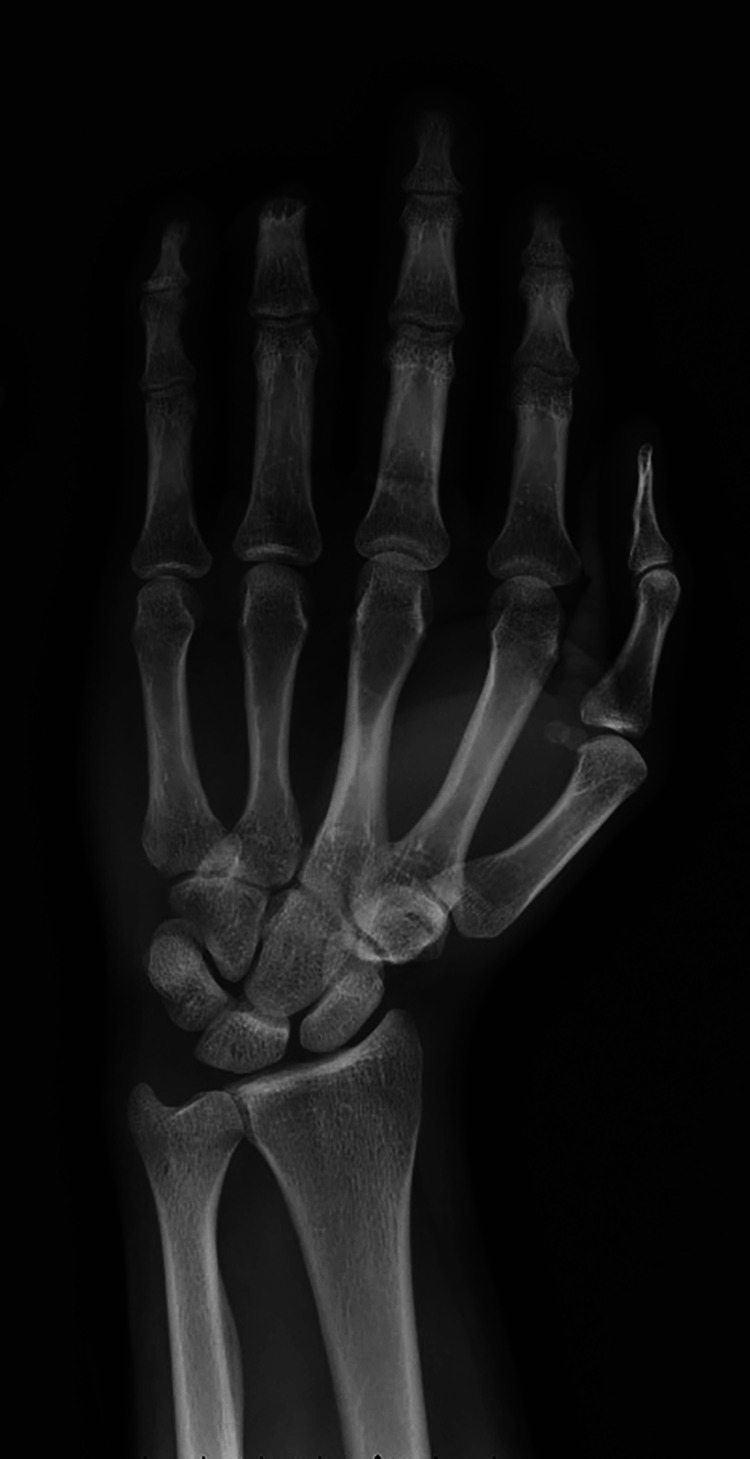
Posteroanterior plain radiograph of the left hand demonstrating amputation through the ring finger middle phalanx.
